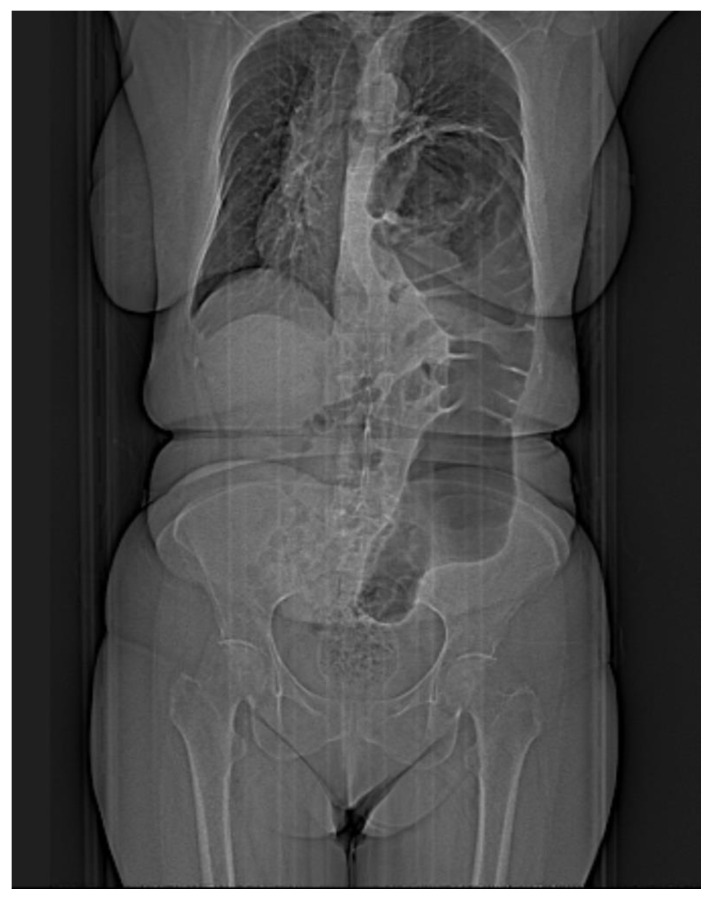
Simple imaging assessment—radiography. The presence of the colon, ascended transdiaphragmatically through a Bochdalek hernia, at the level of the left hemithorax is noted.
